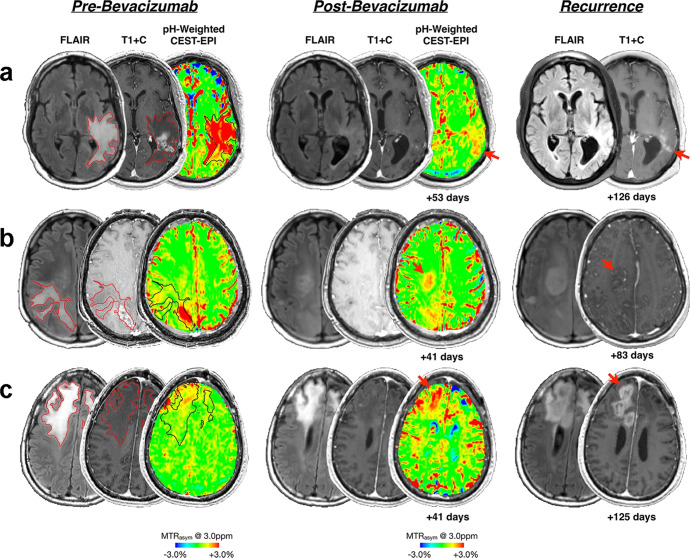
Three patient examples (a: patient #4, b: patient #8, c: patient #11) are demonstrated, with anatomic images (FLAIR and post-contrast T1-weighted images, T1 + C) and pH-weighted CEST-EPI images of MTR_asym_ at 3.0 ppm at three-time points: pre-bevacizumab (baseline), post-bevacizumab (follow-up), and the time of tumor recurrence. Baseline T2 lesion ROIs used for volume and median MTR_asym_ calculation are outlined in red (on FLAIR and post-contrast T1-weighted images) and black (on MTR_asym_ images). The red arrows demonstrate co-localization of residual or newly emerging areas of high acidity (MTR_asym_ at 3 ppm) at the post-treatment time point and the corresponding location of tumor recurrence ~2 months later. CEST-EPI: chemical exchange saturation transfer echoplanar imaging. ROIs: region of interest. FLAIR: fluid-attenuated inversion recovery. (Reproduced from Yao, J. et al. pH-weighted amine chemical exchange saturation transfer echoplanar imaging (CEST-EPI) as a potential early biomarker for bevacizumab failure in recurrent glioblastoma. *J. Neurooncol.*
